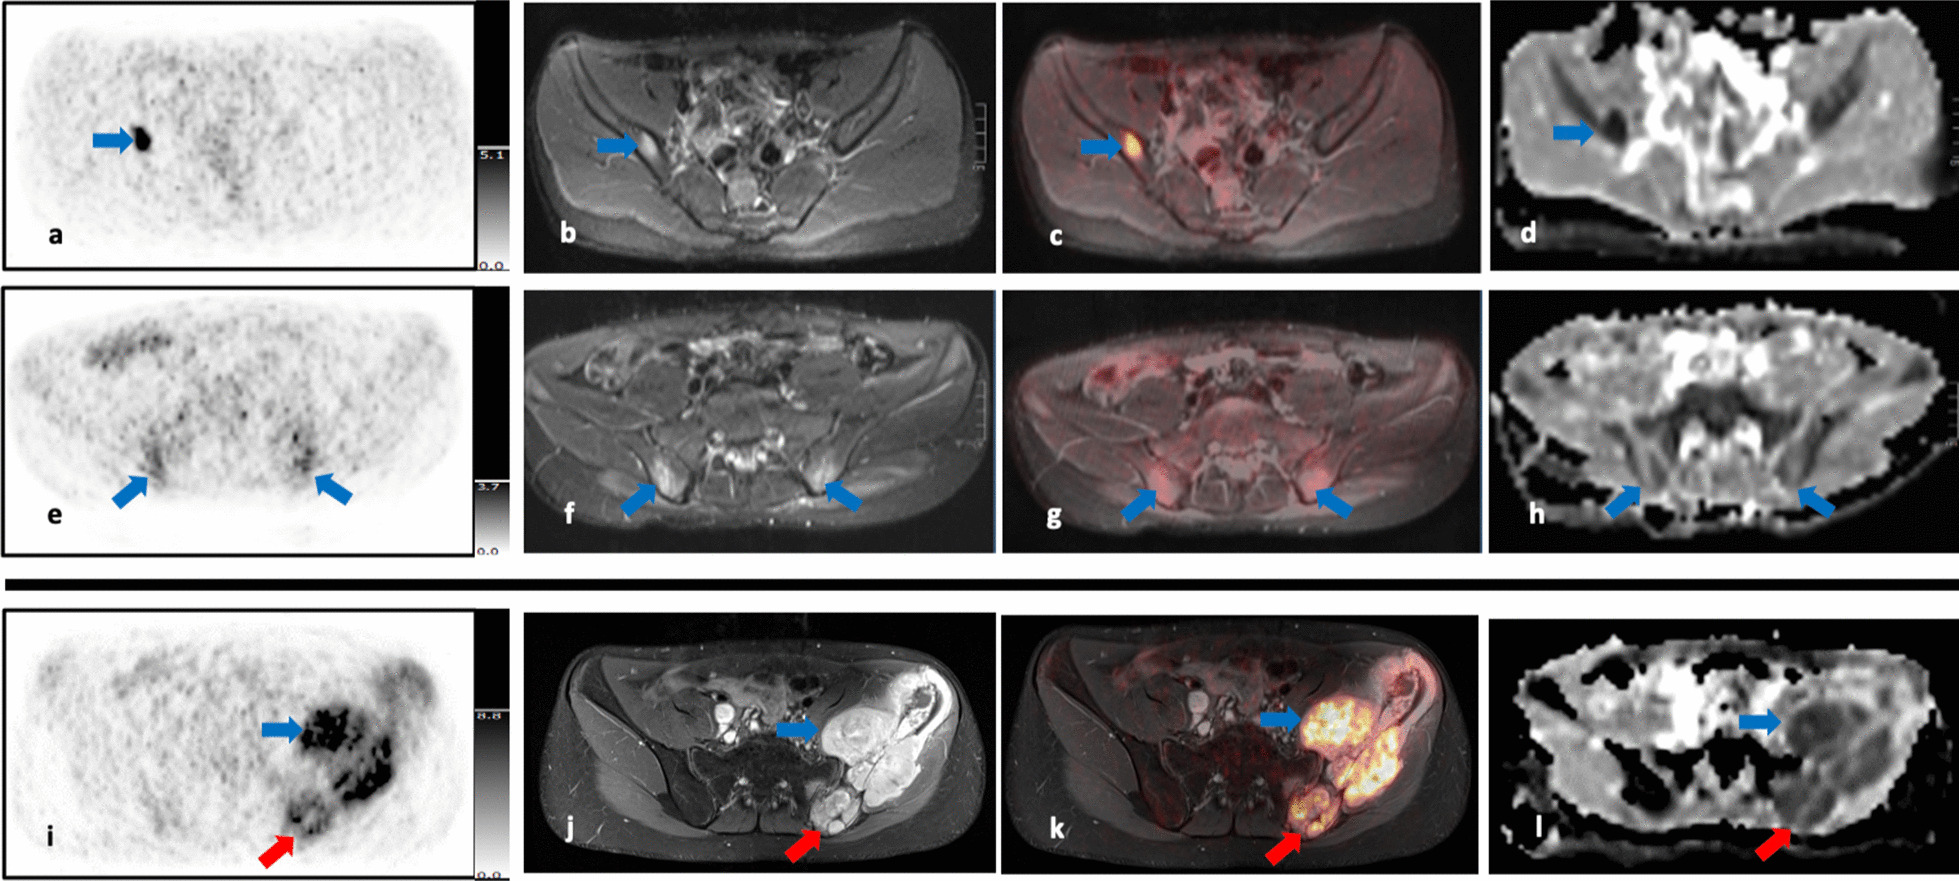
a–l Images from the upper and the middle row belong to the same patient, whereas images from the lower row belong to another patient. The upper row (a–d) shows images of a single lesion in the middle of the right iliac bone (blue arrow). The lesion has increased glucose metabolism on [^18^F]-FDG-PET images (SUV_max_ 9.80) (a, c), is hyperintense on MR T2-TIRM images (b) and has restricted diffusion on DWI (ADC about 419 × 10^–6^ mm^2^/s) (d). The middle row (e–f) shows images including the biopsy sites on both posterior iliac crests (blue arrows): Both biopsy sites have moderate and diffuse radiotracer uptake on [^18^F]-FDG-PET images (SUV_max_ right: 2.65; SUV_max_ left: 2.74) (e,g), are moderately hyperintense on MR T2-TIRM images (f) and do not show restriction in diffusion on DWI (ADC right: about 1240 × 10^–6^ mm^2^/s; ADC left: about 1148 × 10^–6^ mm^2^/s) (h). The third row shows extensive lymphoma involvement of the left iliac bone and the biopsy channel (i-l): Lymphoma manifestation from the left iliac bone have markedly increased glucose metabolism on [^18^F]-FDG-PET images (blue arrows) (i, k), are hyperintense on MR T2-TIRM images (j) and markedly restricted in diffusion on DWI (l). The biopsy channel (red arrows) can be distinguished from the lymphoma lesions by lack of radiotracer uptake (i, k). It is hypointense on MR T2-TIRM images (j) and shows no diffusion restriction on DWI (l)
